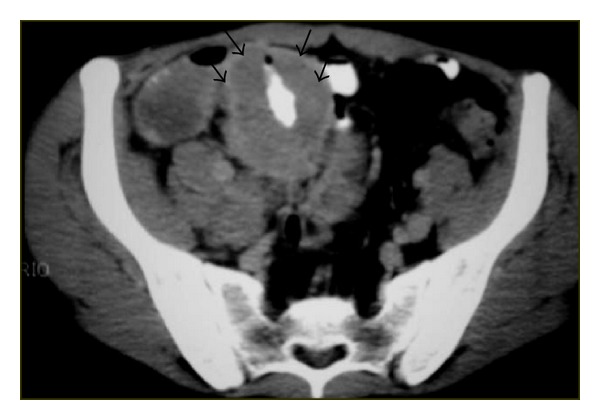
NHL. CT of the pelvis shows concentric thickening of small bowel loop on the right reducing its lumen (arrows).
